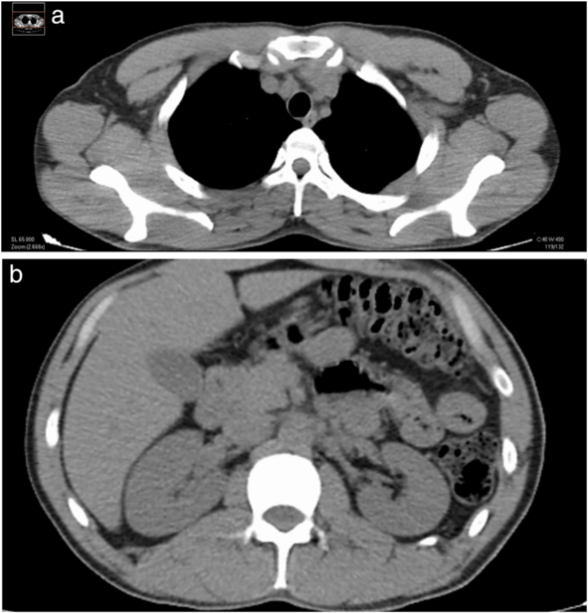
a–b CT scan studies. In CT scan studies, almost no skin retraction at any site is observed. Deep dermis as well as the epidermis seem to be normal again
